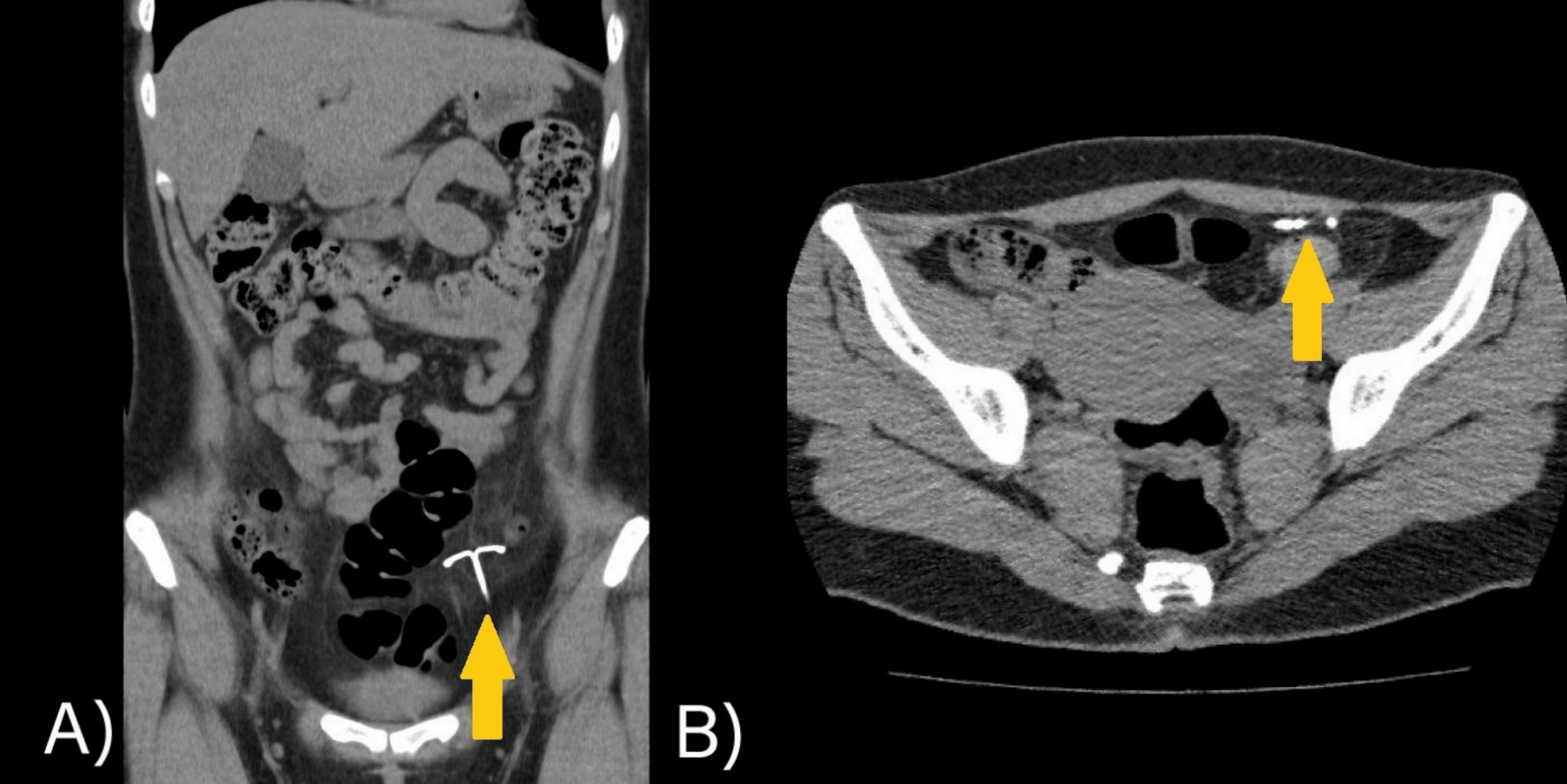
Abdominal CT scan: (A) coronal section and (B) axial section. The yellow arrows show the extrauterine location of the IUD, which is intra-abdominal in the left pelvic hollow. IUD: intrauterine device
